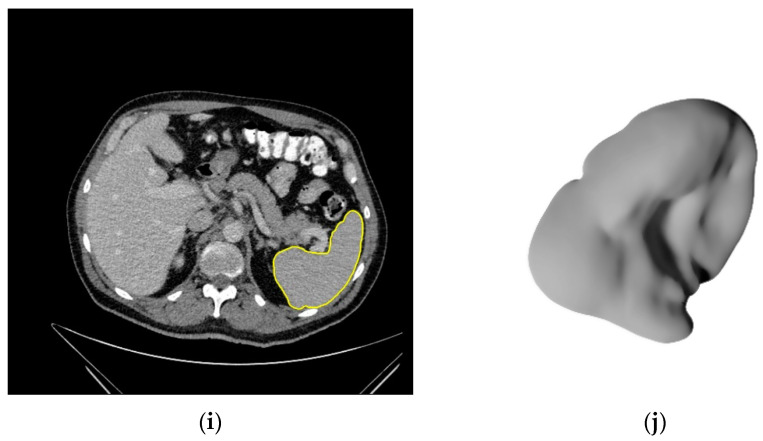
Example CT slices with splenic segmentation and 3D renderings of typical spleens: (a,b) non-lymphoma cohort, (c,d) Hodgkin lymphoma, (e,f) DLBCL, (g,h) follicular lymphoma, (i,j) mantle-cell lymphoma. For each type, we selected the spleen whose radiomics features were closest to the median of all spleens of that type.
